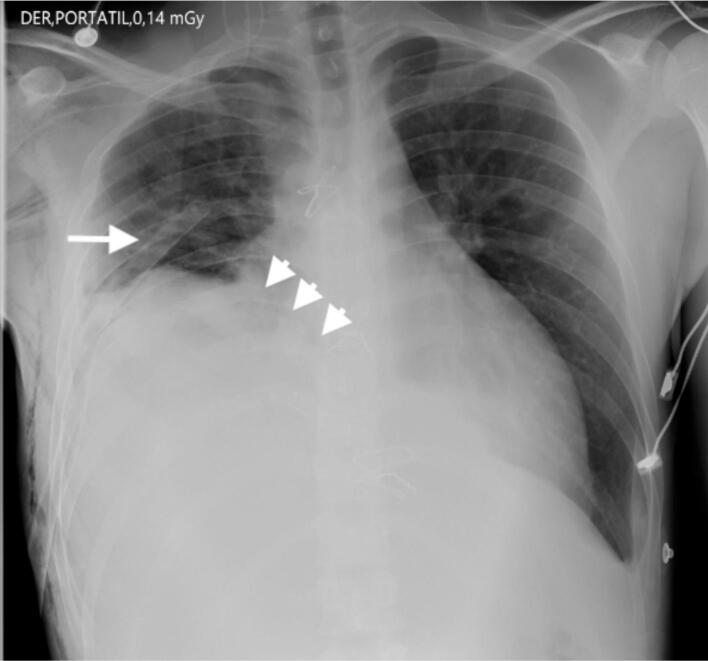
“Chest X-Ray (PA)”. Diffuse opacity in right lung base with lobar atelectasis and pleural effusion (arrowheads) with right diaphragm elevation secondary to a thoracostomy tube (arrow).
